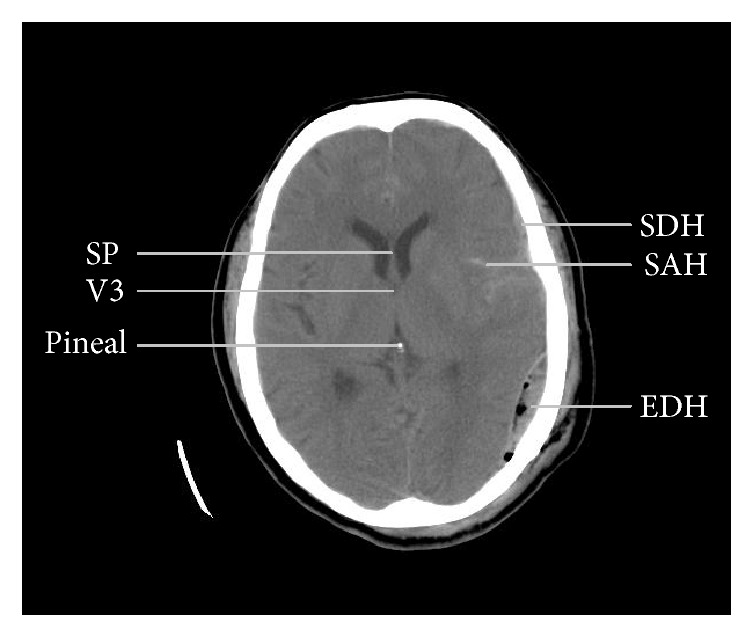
A computed tomographic image from a patient with traumatic brain injury showing anatomical landmarks used to measure midline shift (2 mm in this image) and different types of intracranial hemorrhage. SP: septum pellucidum, V3: third ventricle (only the most rostral part shown), SDH: subdural hematoma, SAH: subarachnoid hemorrhage, and EDH: epidural hematoma.
